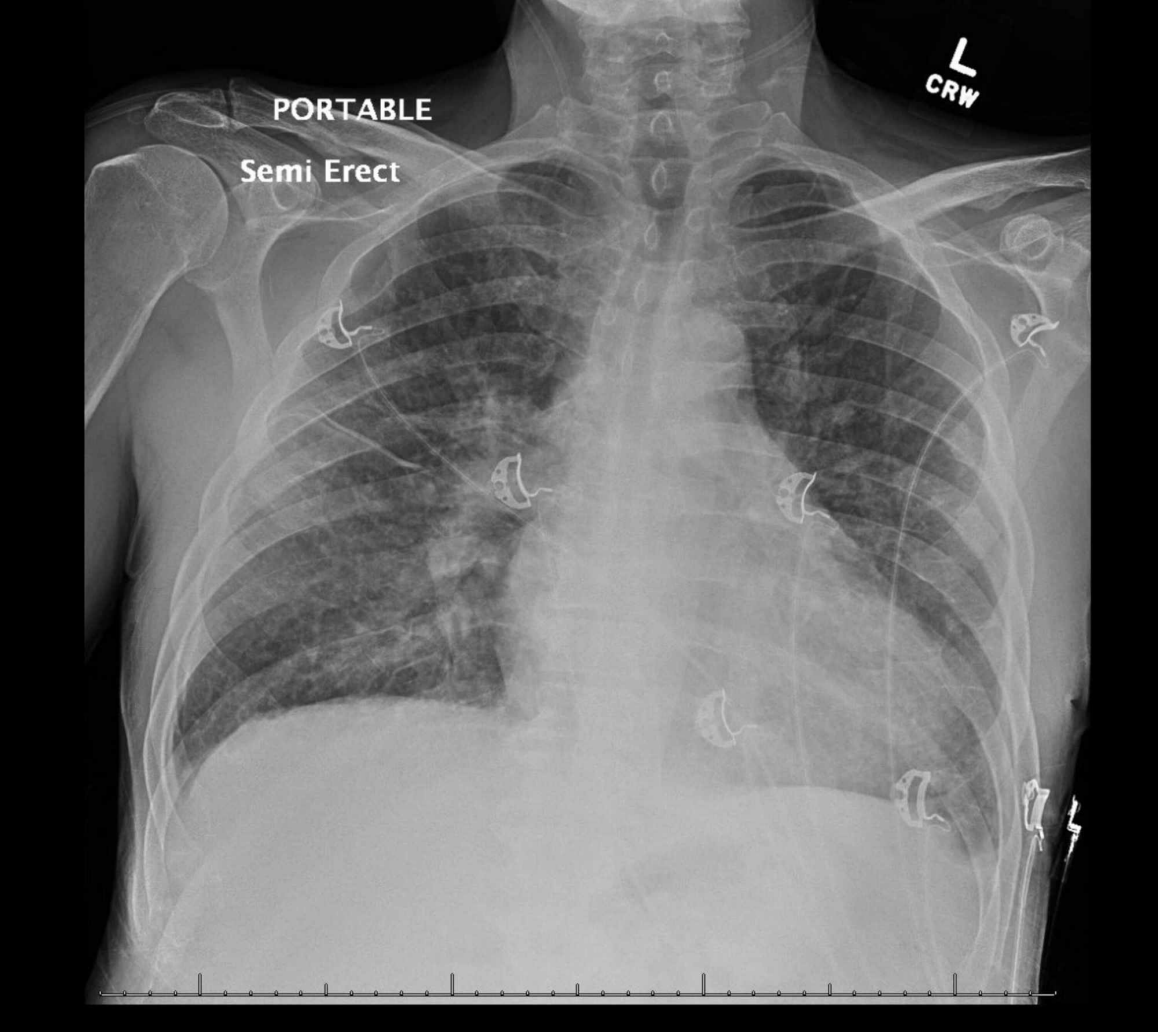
Chest radiograph on admission with mild hypervolemia
